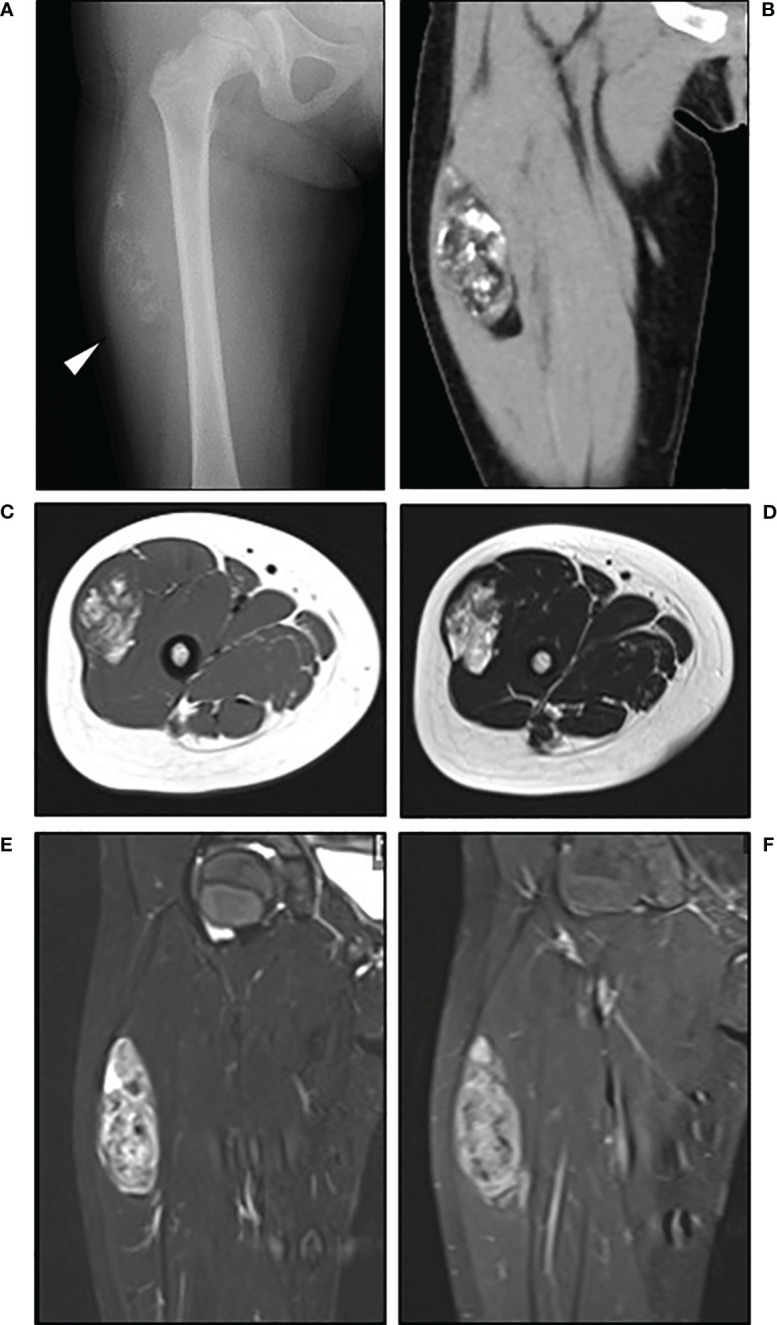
Radiological findings. Plain radiograph (A) showed ossification on the anterolateral thigh (*arrow*) of the patient, a 4-year-old girl. Computed tomography [(B), coronal view] revealed a heterogeneous soft tissue mass with fat attenuation that included nodular ossifications. MRI revealed a relatively well-defined tumor in the right vastus lateralis muscle, with heterogeneous high intensity on T1- [(C), axial view] and T2-weighted imaging [(D), axial view], heterogeneous high intensity on short tau inversion recovery imaging [(E), coronal view], and heterogenous enhancement on gadolinium-enhanced T1-weighed fat-suppressed imaging [(F), coronal view].
